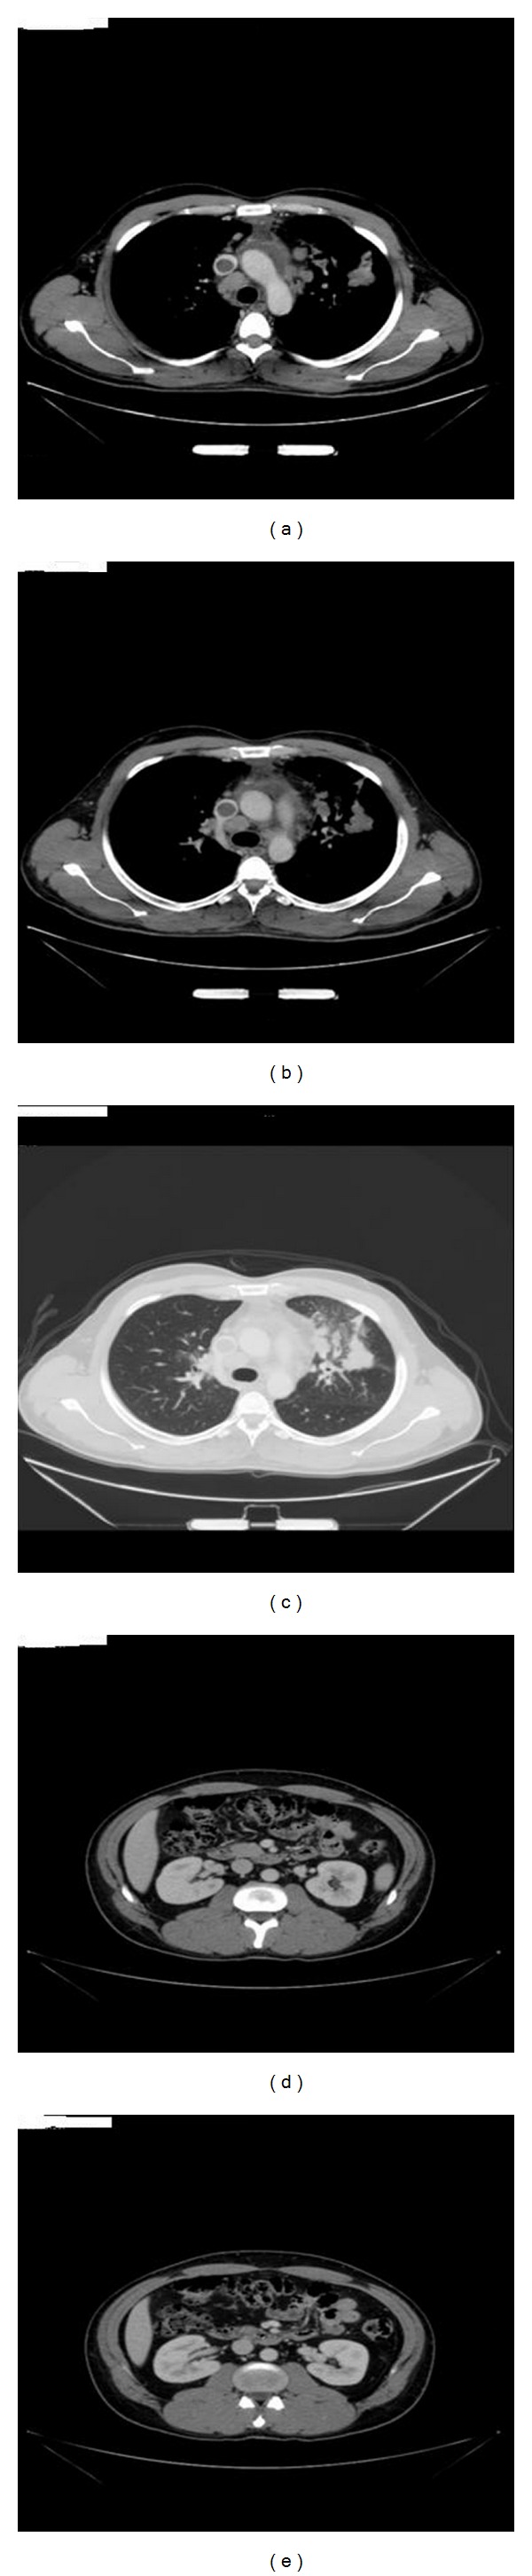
(a, b, and c) CT- chest showed left upper lobe mass mediastinal lymph nodes enlargement and superior vena cava thrombosis.(d and e) CT- abdomen showed spleen, pancreas, both adrenals, and both kidneys are normal. No ascites was noted.
